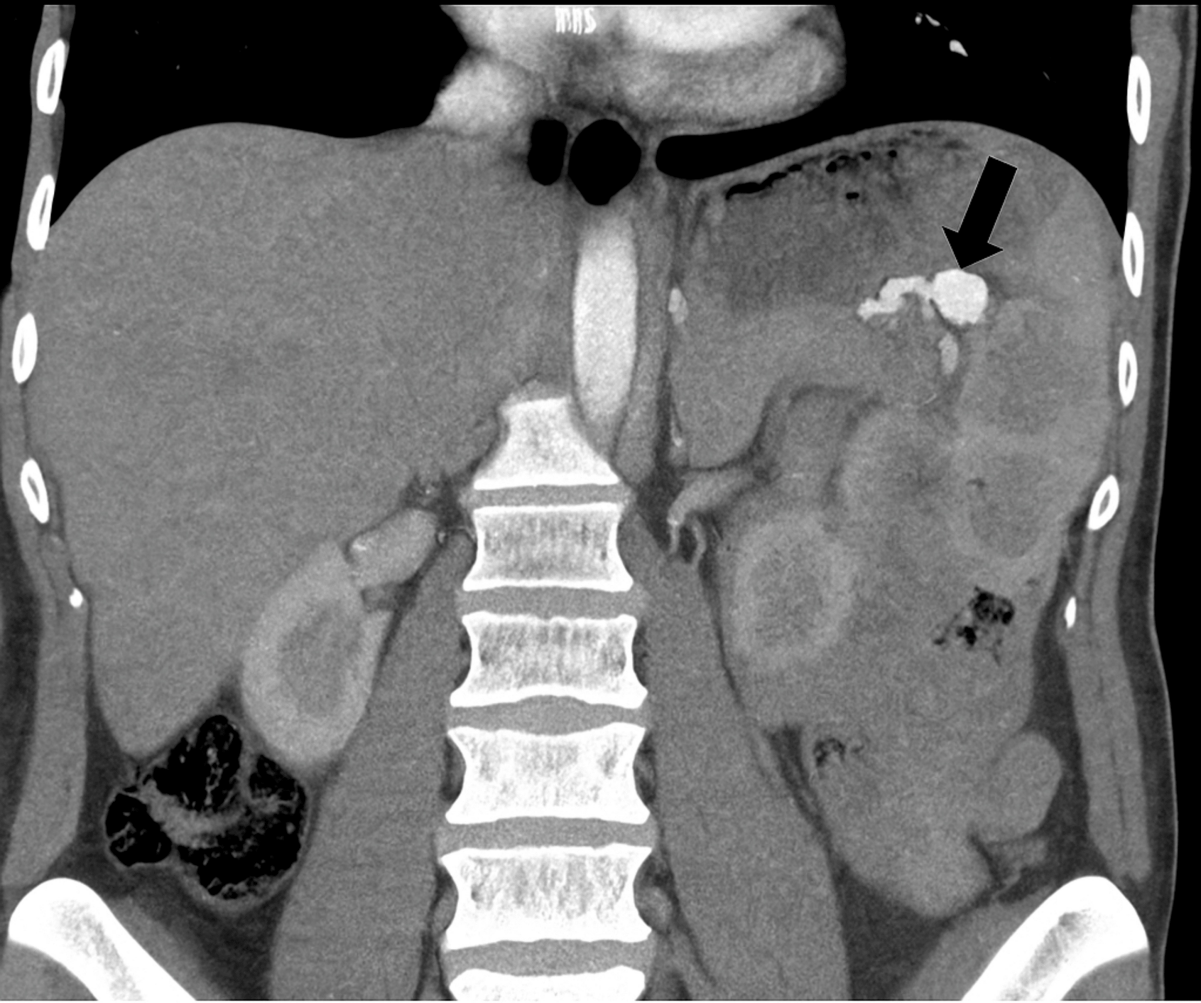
Contrast-enhanced CT angiography showing a 2 cm saccular aneurysm at the splenic hilum.
